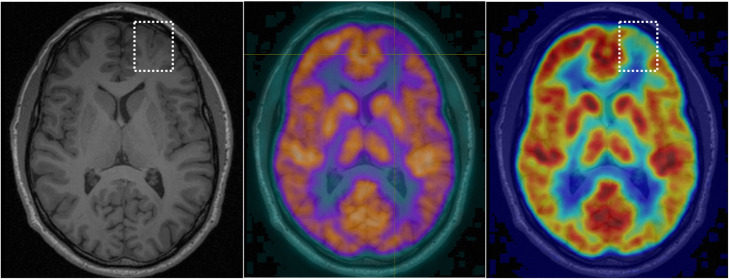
MRI and PET images for an individual with focal PET hypometabolism. A subtle BOSD was detected on MRI in this patient. The structural abnormality is shown in the left image on T1 in the dotted box. ^18^F-FDG PET also showed an area of focal hypometabolism (middle: co-registered PET overlaid on T1 with crosshairs on structural BOSD; right: PET image in jet colour scheme with dotted box on structural abnormality)
